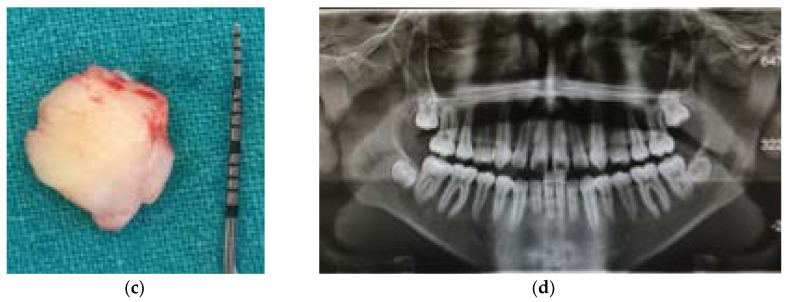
Example of a case treated with operculectomy. (a) Pre-surgical occlusal vision; (b) post-surgical occlusal vision; (c) example of the amount of soft tissue being removed in each side; (d) example of pre-treatment orthopantomography.
